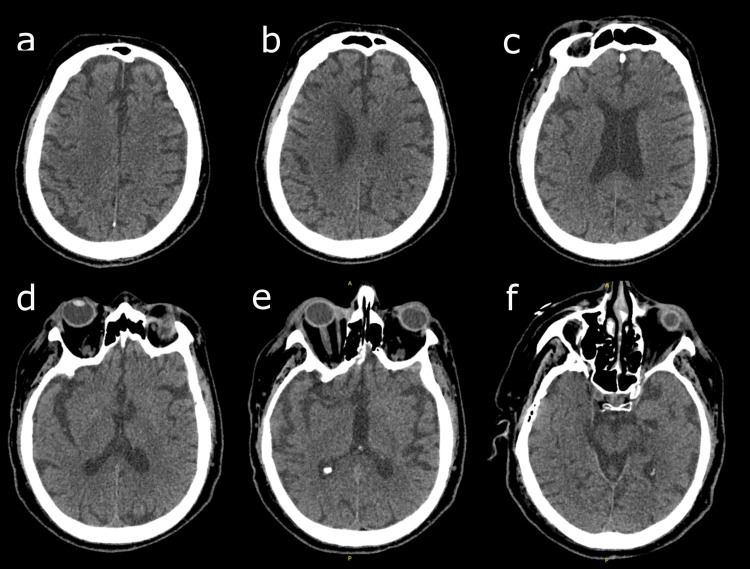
Axial brain CT scan showing no specific abnormality Axial CT scan slices, from top (a) to bottom (f) CT, computed tomography
